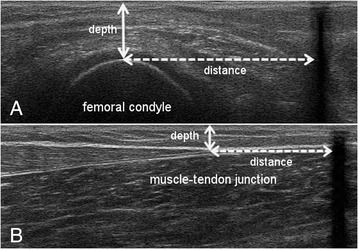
Ultrasound images used to assess the GM muscle belly and Achilles tendon lengths, based on the US-tape method of Barber et al. 2011. The dashed lines show the distance from the superficial aspect of the condyle (a) and the most distal aspect of the muscle-tendon junction (b) to the tape (black shadow), whereby the white arrows indicate the US depth
